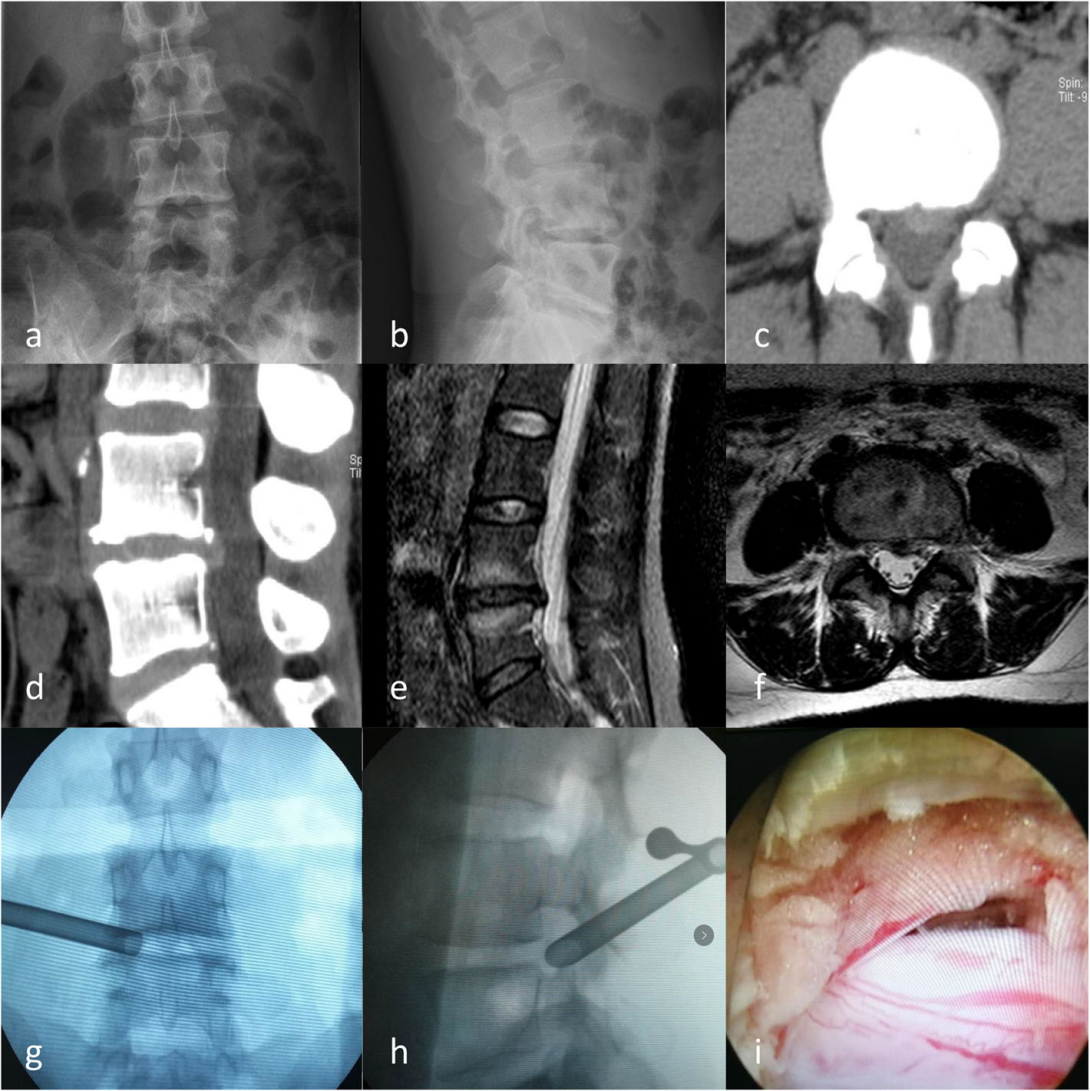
The patient was a 36-year-old female and had a protrusion in the posterior left portion of the L4/5 interverbral discs. a X-ray of anterior and posterior lumbar spine before operation. b Preoperative lateral X-ray of lumbar spine. c In the cross-section, CT revealed a protrusion in the posterior left portion of the L4/5 interverbral discs, which compressed the left L5 nerve root. d CT revealed a left L4/5 interverbral disc protrusion in the sagittal section. e MRI revealed a left L4/5 interverbral disc protrusion in the sagittal section. f In the cross-section, it revealed a protrusion in the posterior left portion of the L4/5 interverbral discs, which compressed the left L5 nerve root. g Placement of the working channel during the operation (anteroposterior film). h Placement of the working channel during the operation (lateral film). i The left L5 nerve root was exposed during the operation
